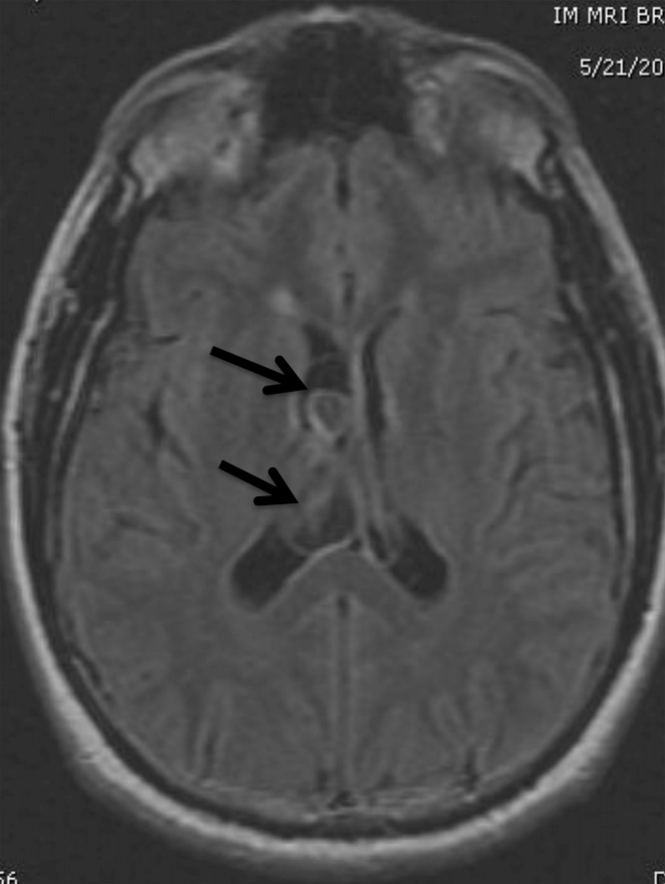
Magnetic resonance imaging of the brain (transverse view) of the patient showing two right intraventricular and one left intraventricular cystic lesions at varying stages. There is a larger 2.3-cm lesion in the posterior aspect of the right ventricle, which follows fluid signal on all sequences. There is subtle mass effect of the right ventricle, which appears asymmetrically enlarged compared with the left ventricle. There is no obstructing lesion in the region of the foramen of Monro or third ventricle. No parenchymal lesions were identified.
